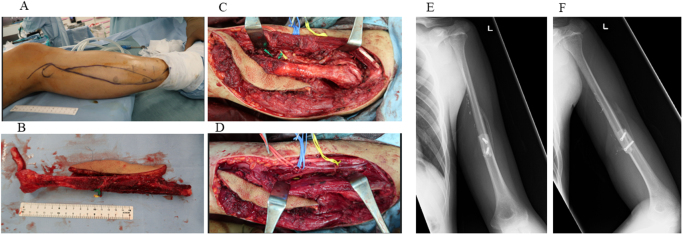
Upper limb reconstruction using a vascularized fibular graft. (A) Right lower leg prior to graft harvesting. (B) Harvested fibular graft with vascular pedicle (donor site). (C) Fixation of the fibula to the recipient site. (D) Microvascular anastomosis between the fibular vessels and the brachial artery and vein. (E) Postoperative anteroposterior radiograph. (F) Postoperative lateral radiograph.
